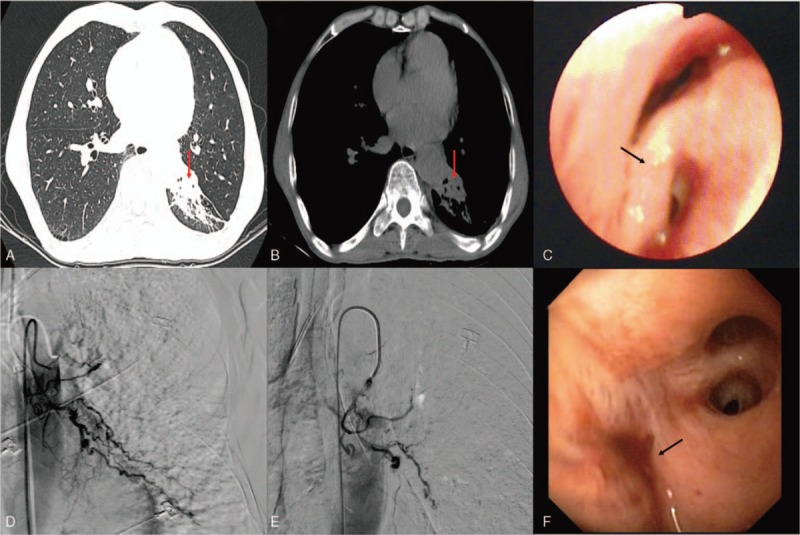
(A and B) Chest computed tomography showing bronchial stenosis in the left lower lobe, accompanied with local atelectasis (red arrow), emphysema, pulmonary bullae, and local thickened pleura. (C) Bronchoscopy showing a slit-like stenosis of the dorsal segment of left lower lobe, edematous, smooth mucosa, and widening of carina (black arrow), no abnormal vessels or active bleeding is seen. (D) Selective bronchial arteriogram showing a dilated, tortuous left lower lobe bronchial artery and profusely hypervascularized dorsal segment of left lower lobe. (E) Transcatheter embolization of the hypertrophic bronchial artery using poly-vinyl alcohol particles (PVA) 500 μm in diameter. After embolization, DSA reveals complete disappearance of the abnormal artery. (F) The opening of left lower bronchial lobe is occluded, but there is no active hemorrhage after BAE (black arrow).
